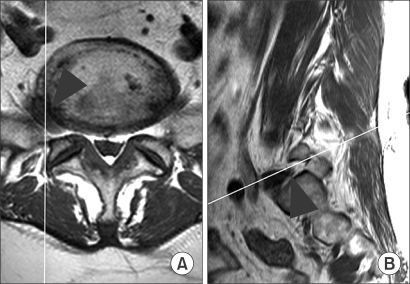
The axial (A) and sagittal (B) MR images of a 58 year-old woman are shown. The white line in each figure indicates the sectioning plane for the other figure. An extraforaminal herniation on the right side at L5-S1 (arrowhead) was suspected from the axial image (A). The sagittal image obtained in the extraforaminal zone (arrowhead) was not helpful due to the lack of reliable anatomical landmarks (B). These images led to different interpretations among the three observers during both reading sessions, i.e., positive, negative, negative during the first, and positive, negative, and positive during the second. This patient achieved symptom improvement by a L5 root block; no further imaging study was done.
